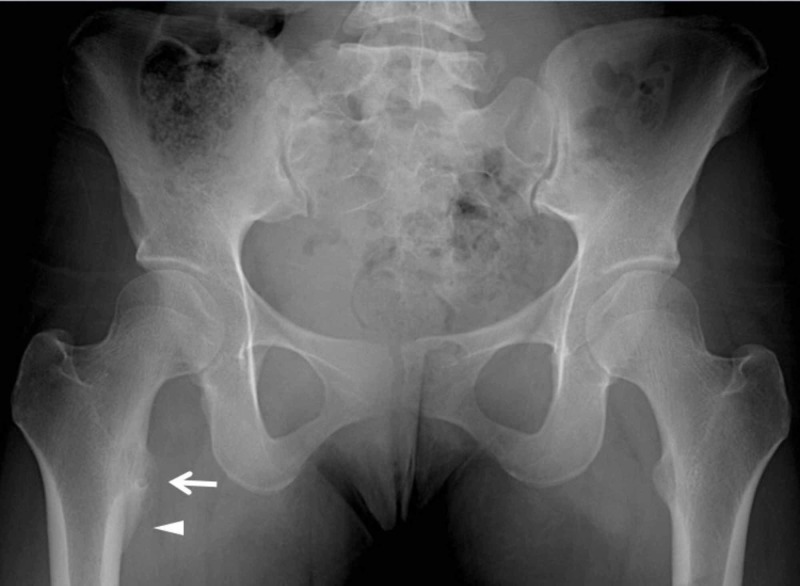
Anterior-posterior radiograph of the pelvis obtained five months after the symptom onset. A 16-year-old female with a lytic lesion of the right femoral lesser trochanter cortex. Anterior-posterior radiograph of the pelvis obtained five months after the symptom onset shows a lytic lesion (arrow) with indistinct margins and no definite nidus. There is associated smooth periosteal reaction (arrowhead).
